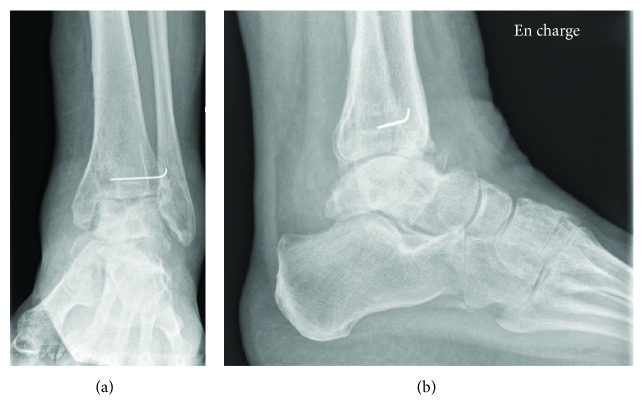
(a) AP and (b) lateral X-rays of the left ankle at 1 year, showing necrosis of the talus and pilon, with anteromedial articular conflict.
